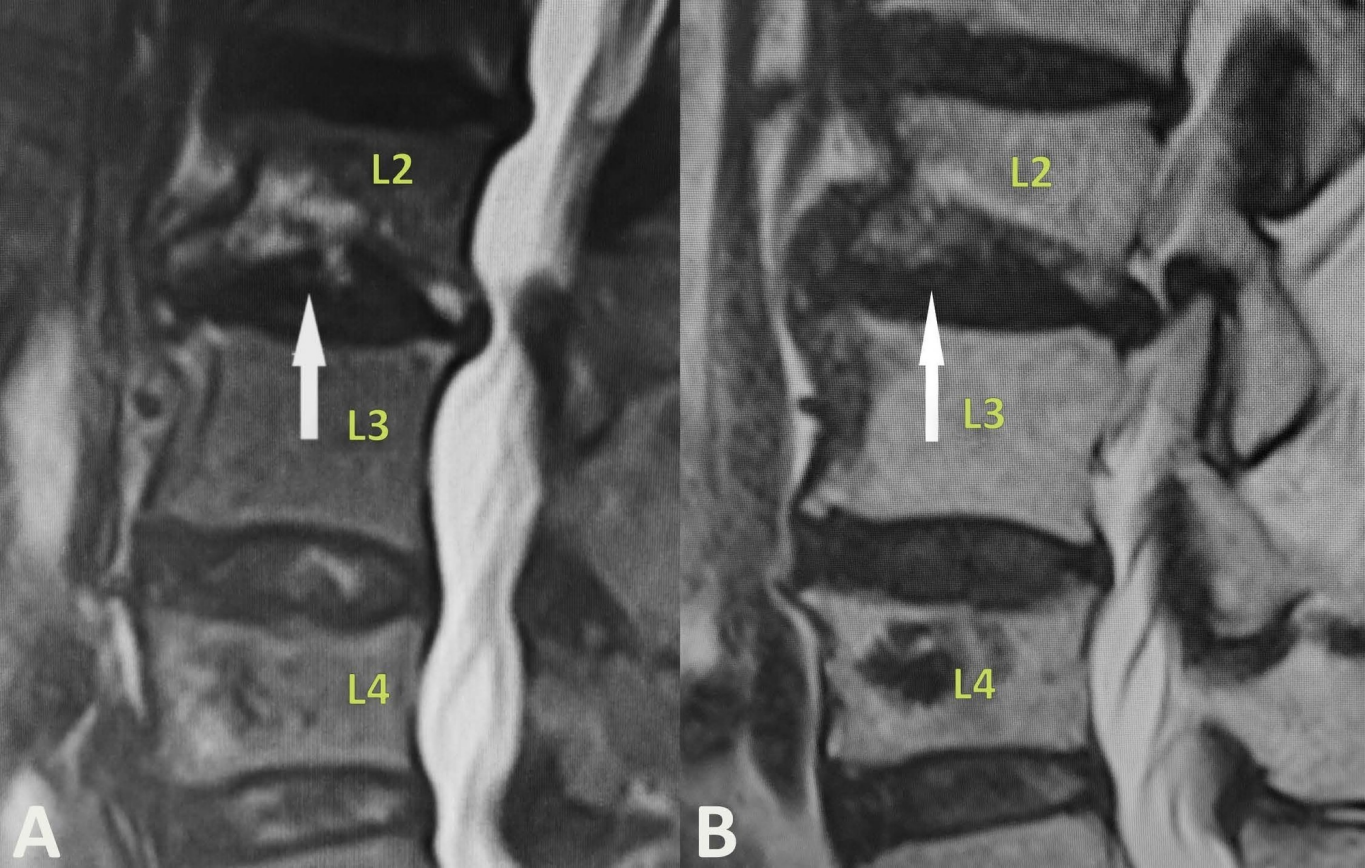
Sagittal short-TI inversion recovery (A) and standard T2-weighted (B) MRI scans show an acute L2 compression fracture with inferior endplate involvement
